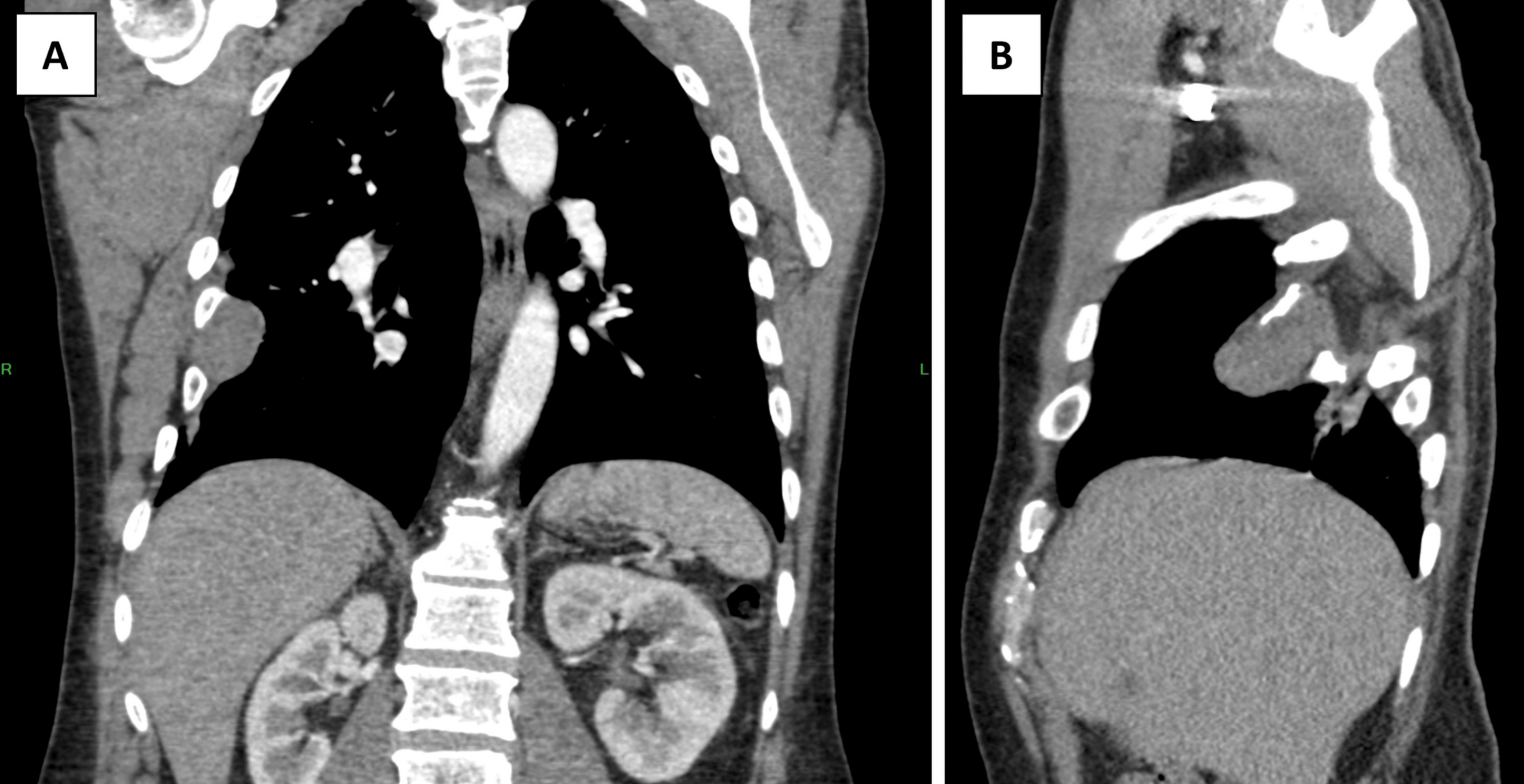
Pre-operative CT scan of the schwannoma to identify anatomical location CT: Computed tomography; A: Coronal plane; B: Sagittal plane
